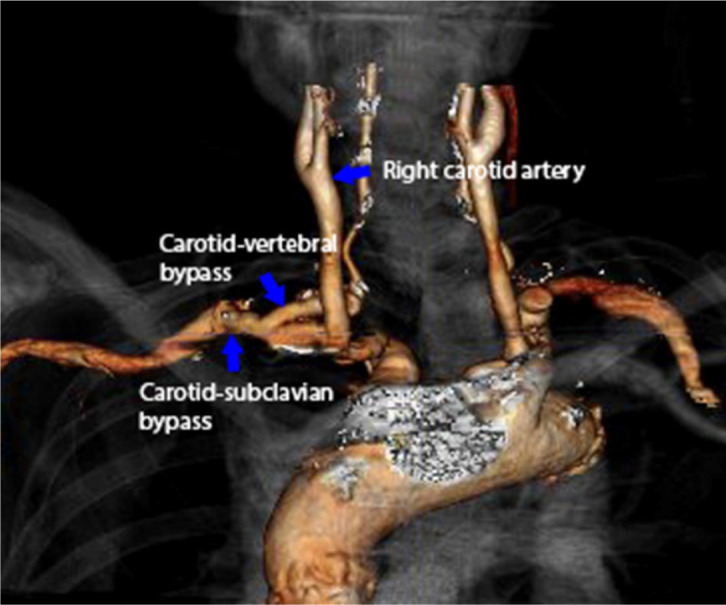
Postoperative computed tomography angiography with three-dimensional
